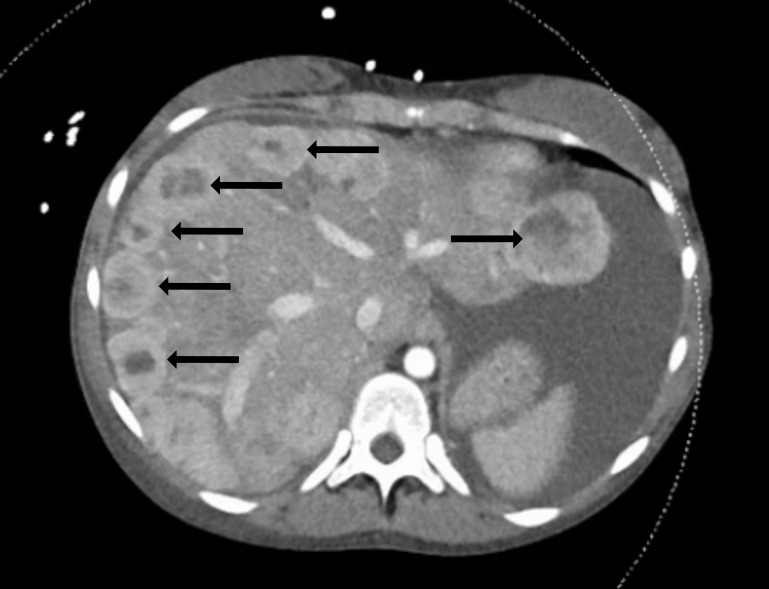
Computed tomography axial view with intravenous contrast of the abdomen of a 60-year-old female with bilateral lower extremity swelling. Multiple hepatic lesions are demonstrated (white arrows).
